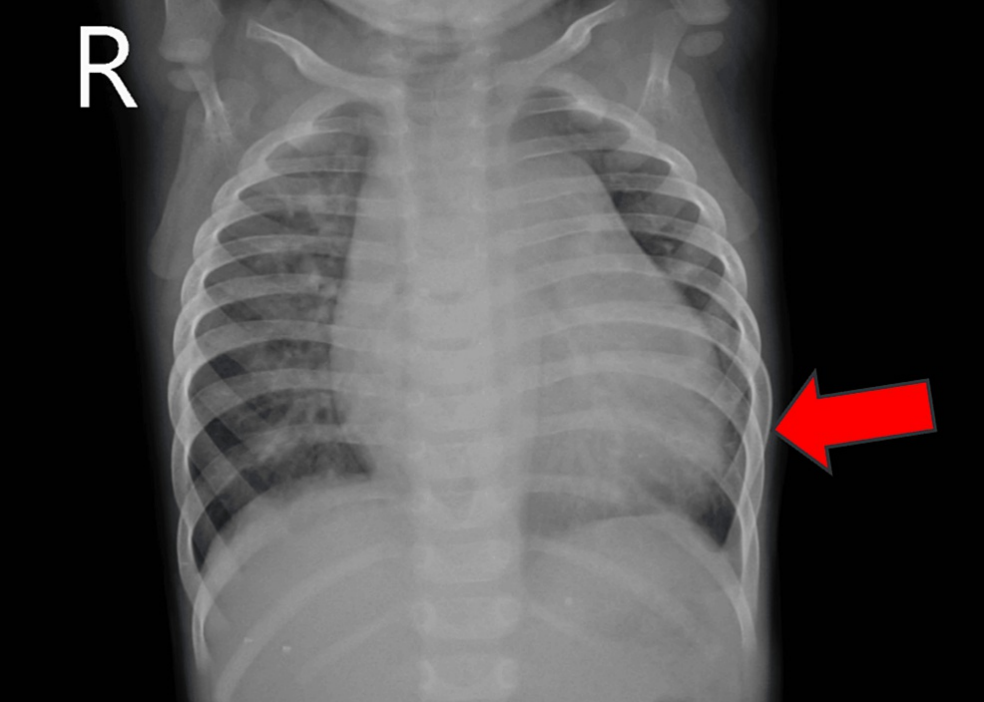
A chest X-ray showing cardiomegaly (red arrow)
